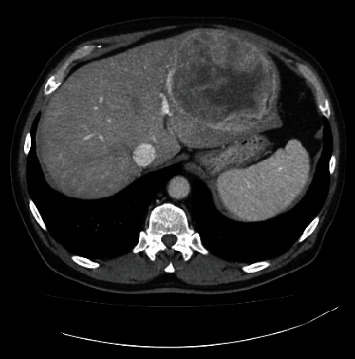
Contrast-enhanced abdominal computed tomography (CT) showed a large well-circumscribed mass in the lateral segment of the left hepatic lobe with early arterial phase enhancement.
